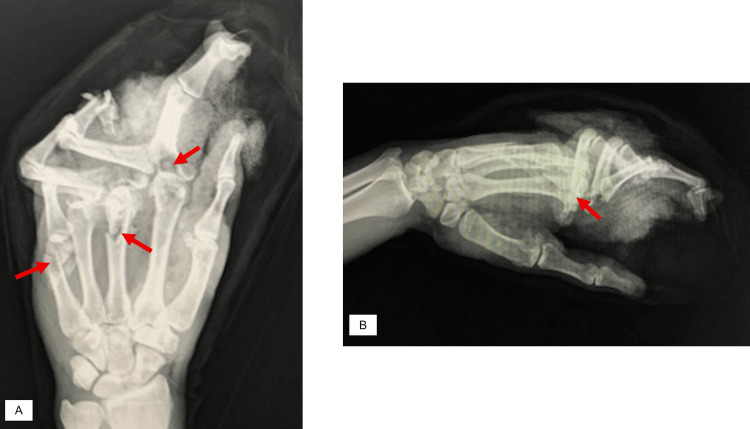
Preoperative radiographs of the left hand The preoperative injury radiographs demonstrate multiple fractures of the metacarpals and proximal phalanges (A and B) (red arrows).
